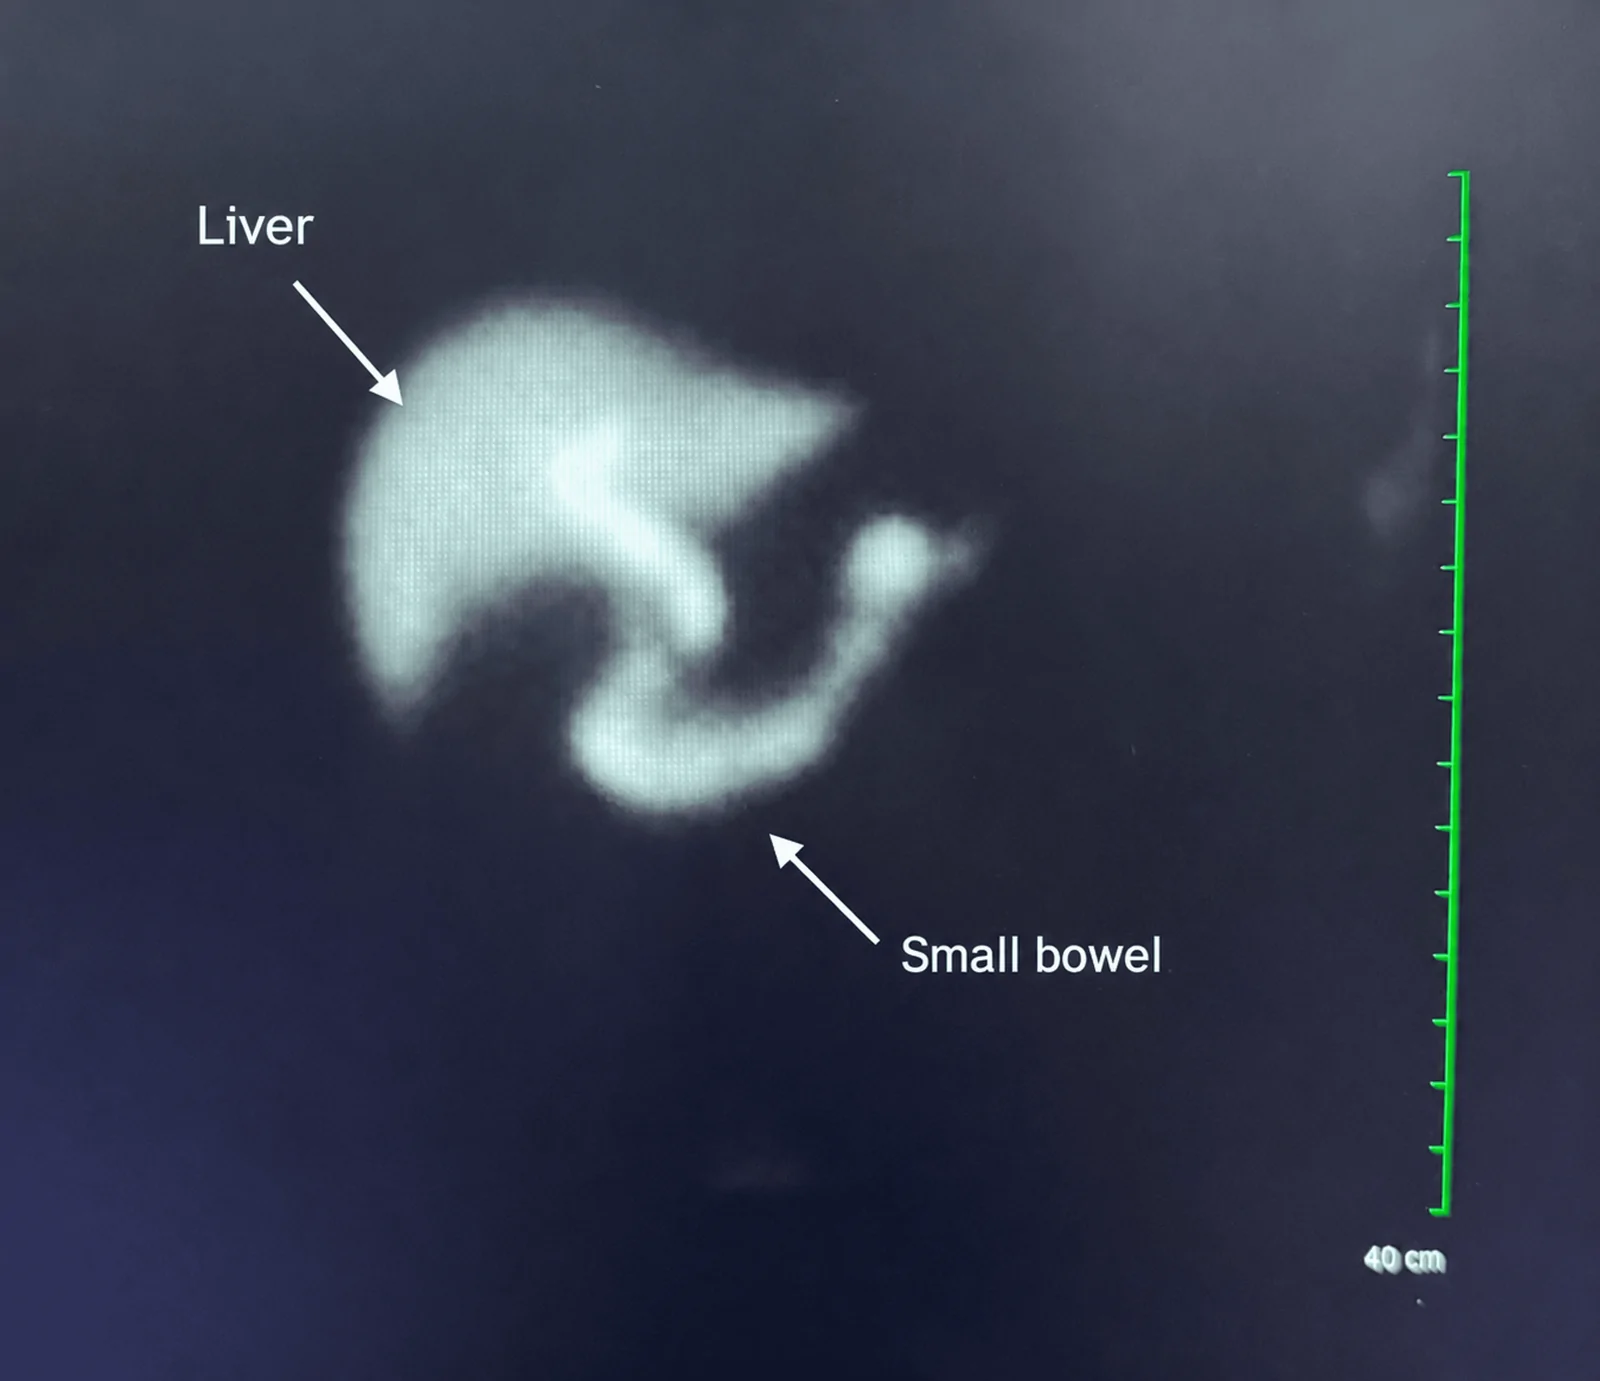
Hepatobiliary scintigraphy (HIDA scan) Prominent radiotracer uptake is seen within the liver parenchyma (indicated by the Liver arrow) and radiotracer passage into the small bowel (indicated by the Small bowel arrow). The complete HIDA examination was interpreted as showing no evidence of acute cholecystitis. HIDA, hepatobiliary iminodiacetic acid
